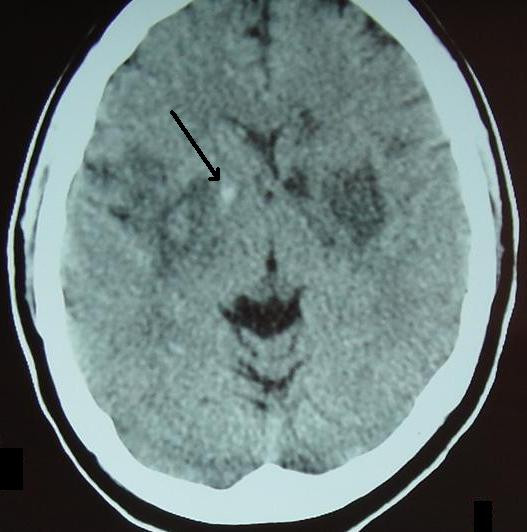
Non contrast brain CT scan of our patient at the time of admission. Note the bilateral hypodensities, which fit the area of the lenticular nucleus (putamen and globus pallidus) on both sides. There is also small hyperdensity at the right globus pallidus (black arrow). This prompted the physician to suspect an infectious process instead of a vascular one. Our patient had bilateral infarction of the lenticular nucleus with early hemorrhagic transformation inside the right one.
